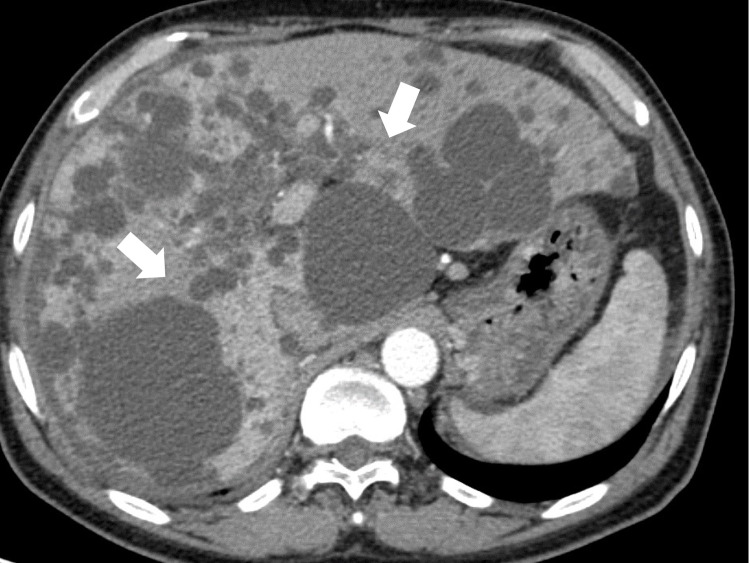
Enhanced computed tomography of the liver two months prior to the patient’s admission. Several cysts (white arrows) are visible in the liver; however, no infectious finding is detected.
